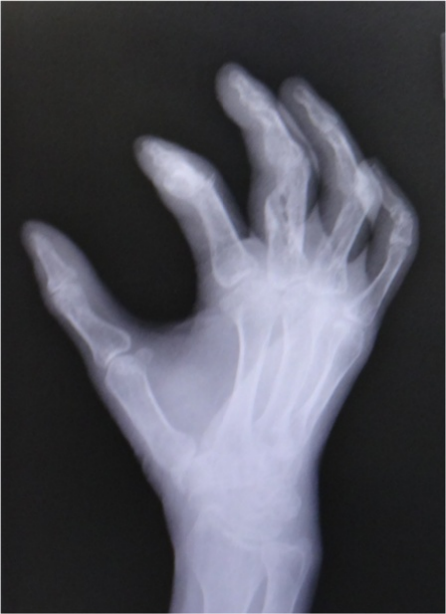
An X-ray image 15 months after surgery shows bone union of PIP joint of index finger
